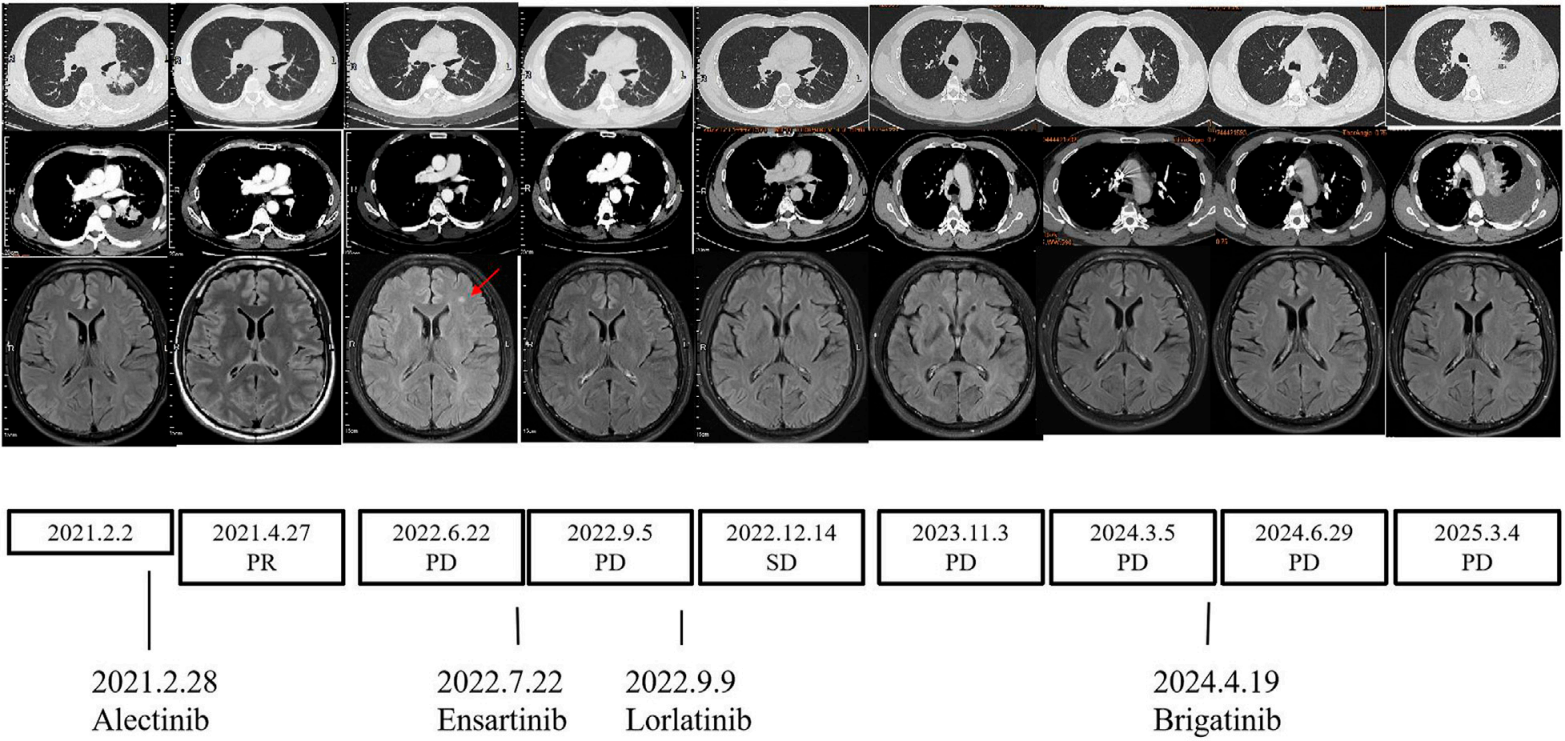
Changes during therapy. CT scans, MRI scans, and treatment timeline. CT, chest computed tomography; MRI, magnetic resonance imaging.
